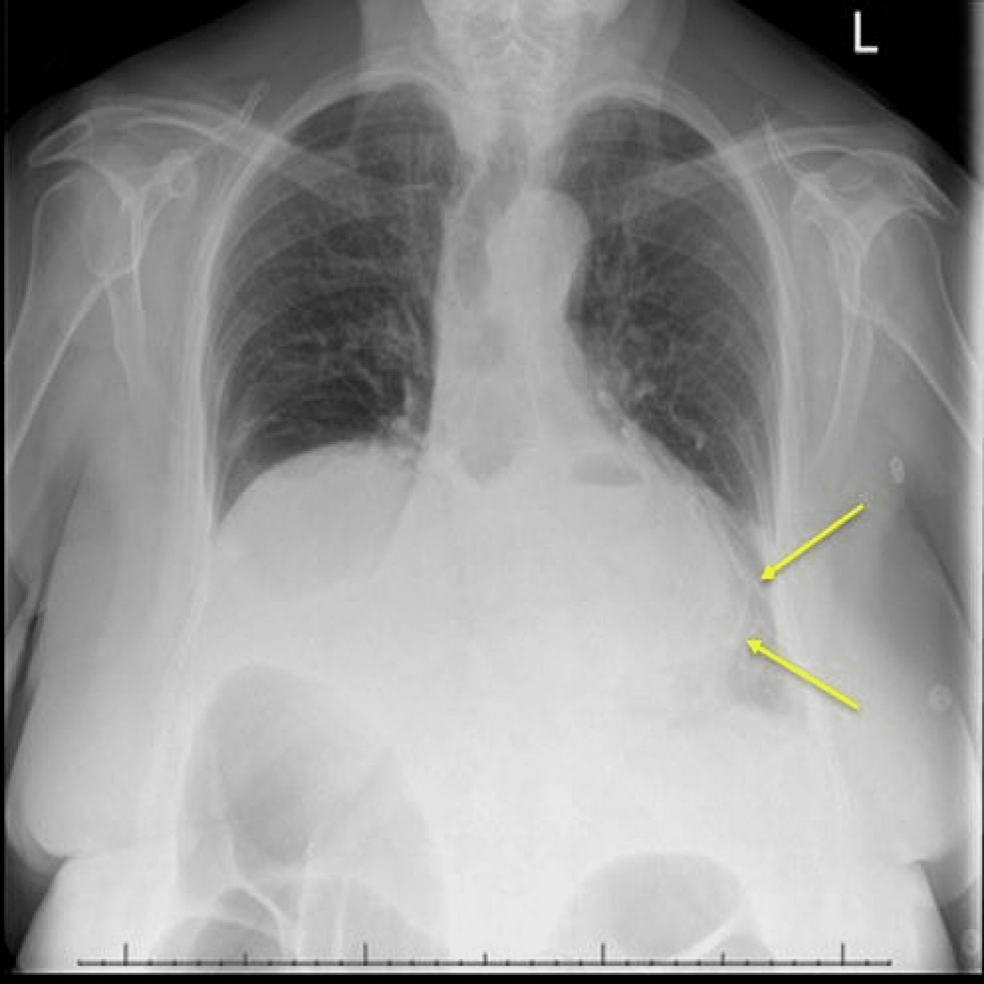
Chest x-ray with air fluid levels (yellow arrows).
